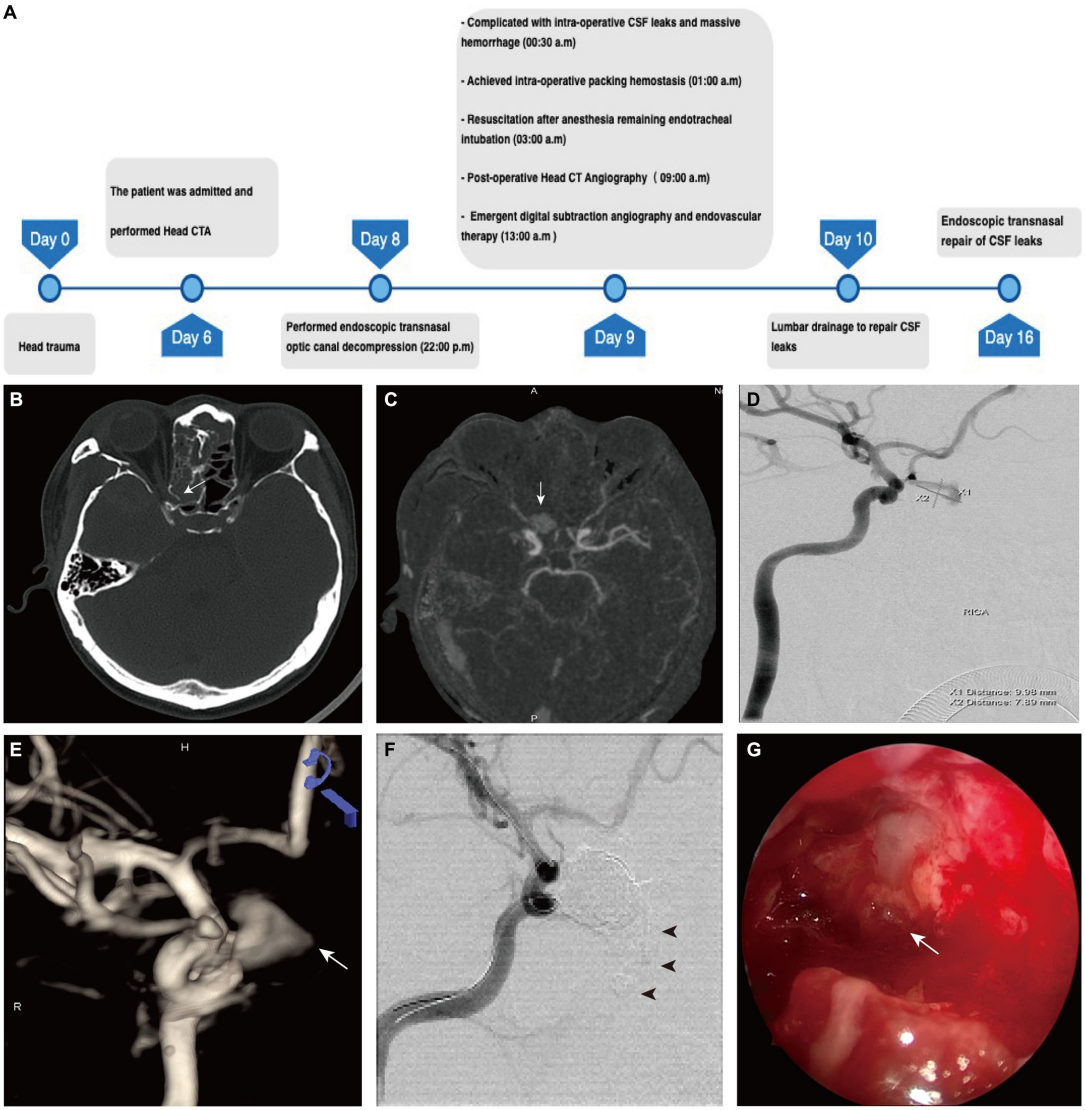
The preoperative evaluation and surgical process in Case 1. (A) The timeline of management in case 1. (B) Preoperative CT of the head demonstrates lateral wall of sphenoid sinus cavity that protrudes into the optic canal (*arrow*). (C) Postoperative CTA shows enhancement of the central patent portion of the pseudoaneurysm that protrudes into the sphenoid sinus cavity (*arrow*). (D) A pseudoaneurysm is visualized arising from the ophthalmic artery in the early arterial phase of ophthalmic artery after contrast injection. (E) 3D reconstruction of DSA demonstrates the irregular pseudoaneurysm with the feature of re-rupture (*arrow*). (F) The angiogram obtained after endovascular treatment demonstrates complete occlusion of the pseudoaneurysm. Onyx was infused into the ethmoid sinus cavity (*arrowhead*). (G) The surgical field of cerebrospinal fluid leak repair demonstrates the residual pulsation of embolized pseudoaneurysm (*arrow*).
